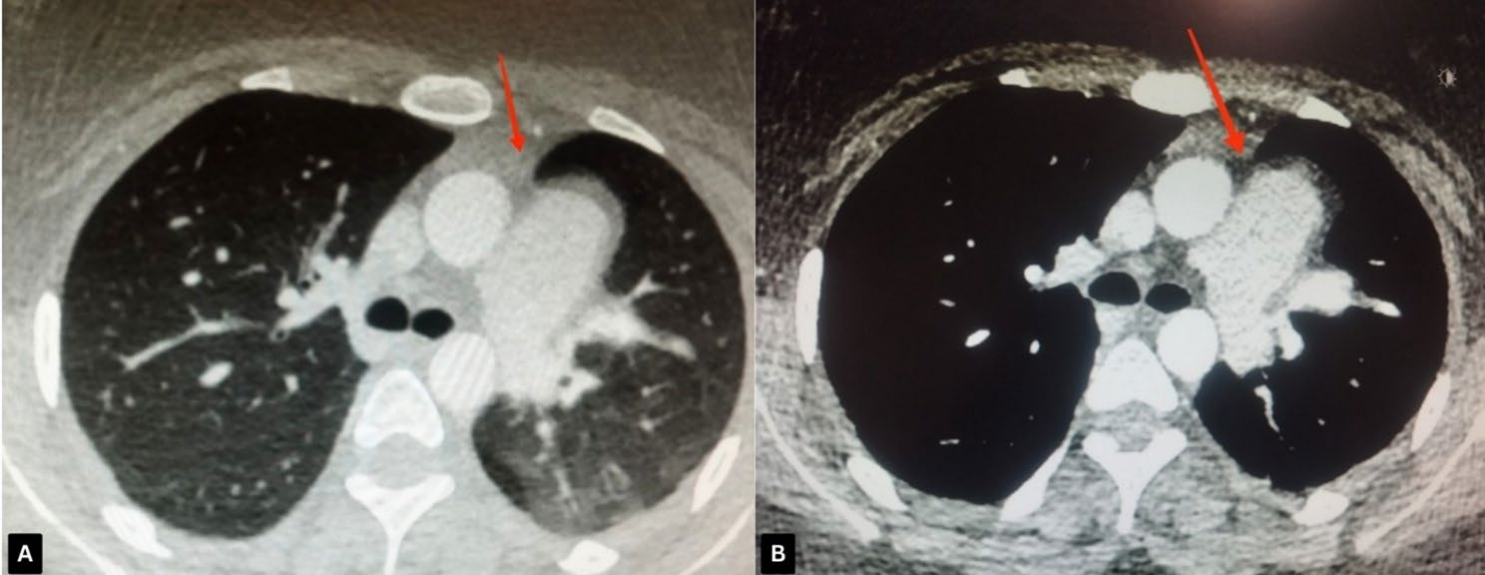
Chest CT Scan (Axial View): Lung tissue is detected between the aorta and the main segment of the pulmonary artery (red arrow). Mosaic attenuation is observed in the upper lobe of the left lung (A and B)
